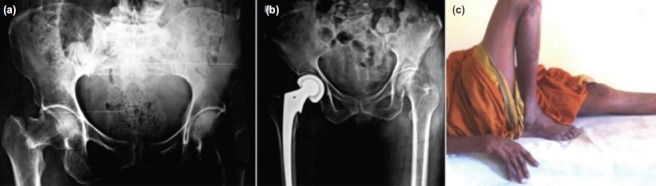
(a) Pre and (b) Post op radiographic images of patient treated with total hip replacement for femur neck fracture. (c) Clinical picture of patient after 1 year of treatment.
